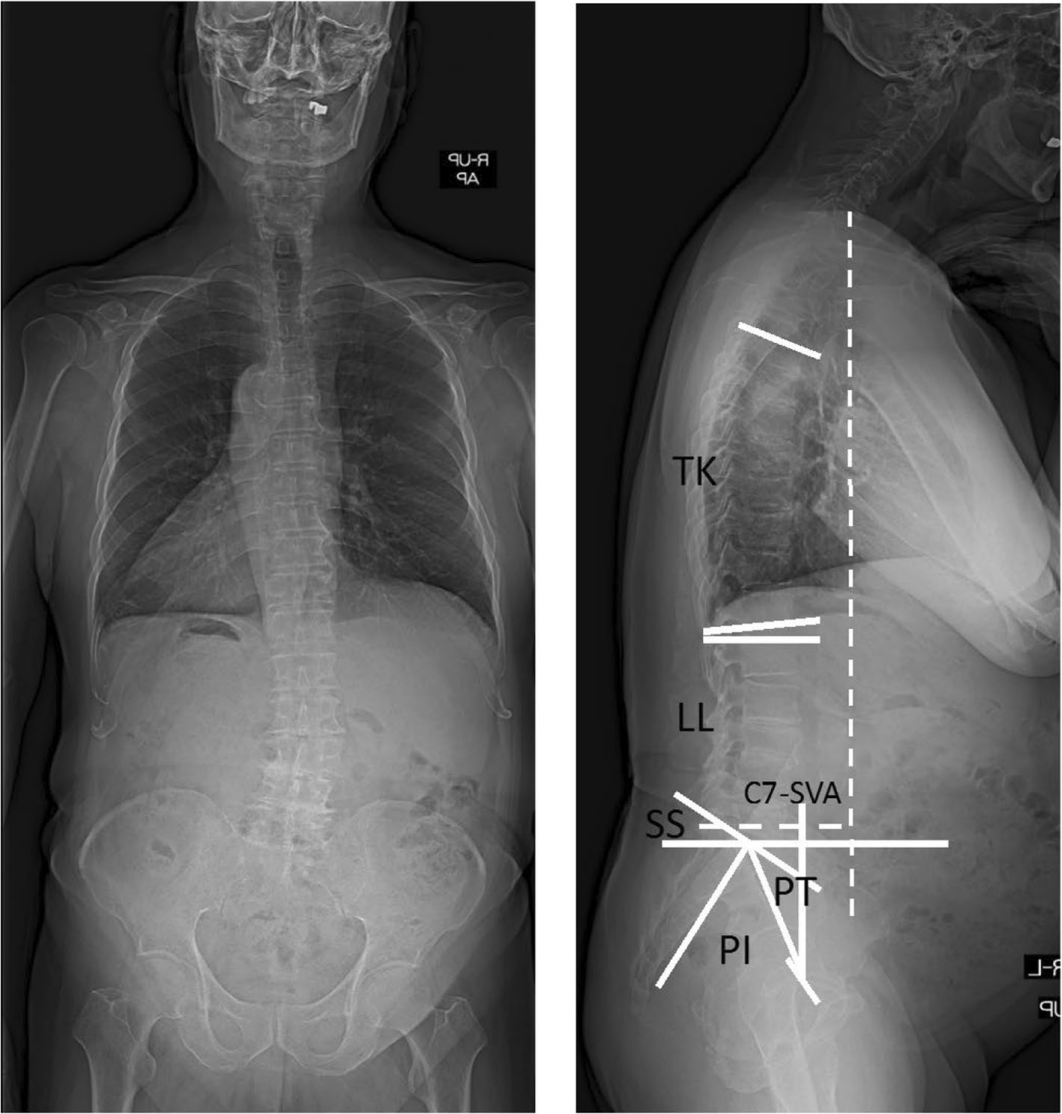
Standing-erect whole-spine posteroanterior and lateral full-spine radiographs. Measurement of sagittal parameters. Thoracic kyphosis (TK) was the Cobb’s angle between upper end plate of T5 and lower end plate of T12. Lumbar lordosis (LL) was the Cobb’s angle between upper end plate of L1 and S1. Pelvic incidence (PI) was the angle between the perpendicular to the sacral plate at its midpoint and the line connecting this point to the middle axis of both femoral heads. Pelvic tilt (PT) was the angle between the line connecting the midpoint of the sacral plate to the axis of the femoral heads and the line perpendicular to the floor. Sacral slope (SS) is defined as the angle subtended by the horizontal line and upper sacral end plate. Sagittal vertical axis (C7-SVA) was the distance between the C7 plumb line and the postero- superior corner of S1
